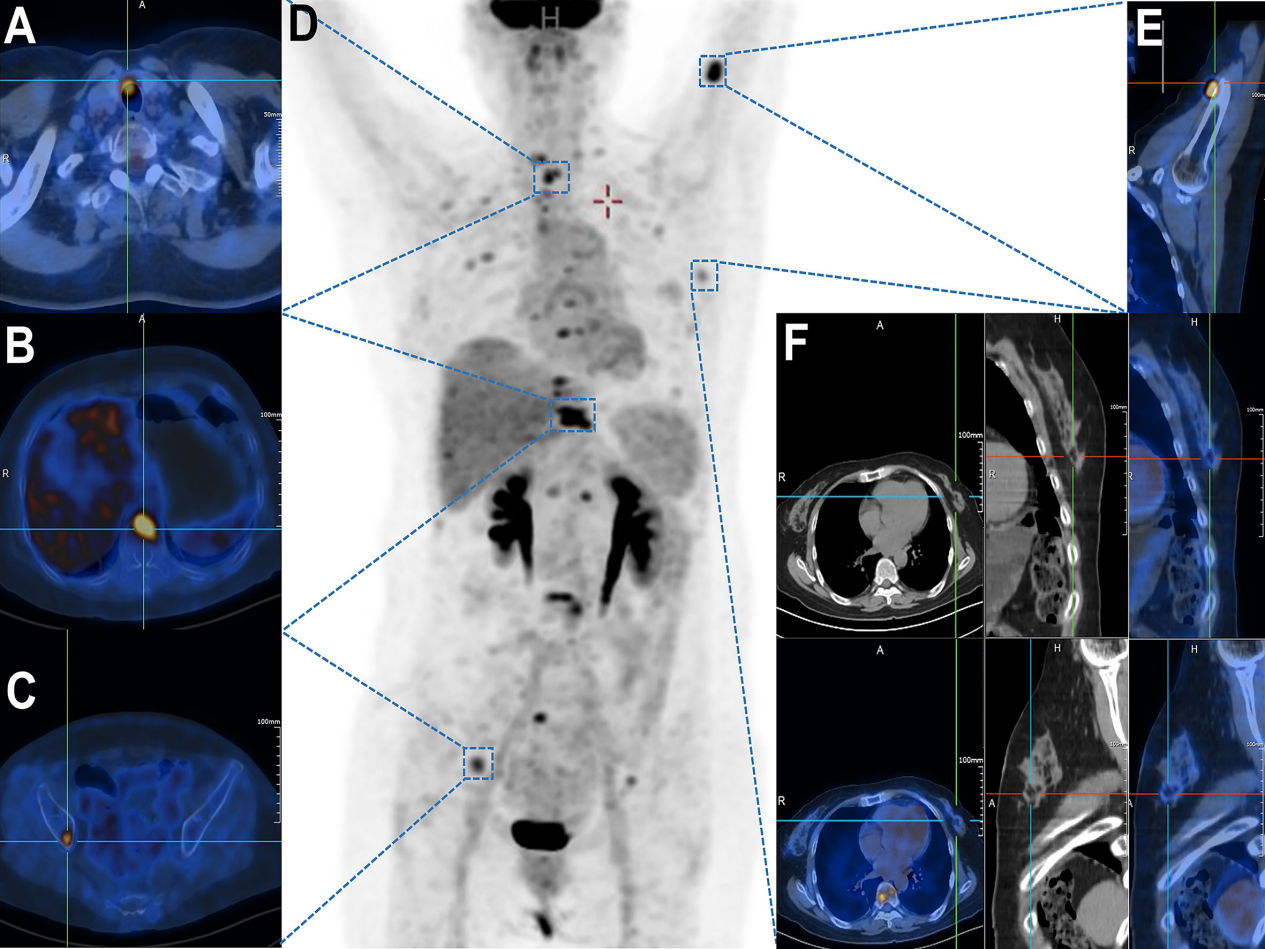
Whole-body ^18^F-FDG PET/CT demonstrating multifocal hypermetabolic lesions. (A) Thyroid isthmus lesion with focally increased FDG uptake (SUVmax = 9.41). (B) Vertebral metastasis at T10 level (SUVmax = 11.64). (C) Right iliac bone lesion showing elevated metabolic activity (SUVmax = [*value*]). (D) Maximum intensity projection (MIP) image revealing systemic dissemination of metabolically active metastases. (E) Left proximal humeral lesion with abnormal FDG avidity (SUVmax = [*value*]). (F) A suspicious mass in the left breast upper-outer quadrant demonstrated focal FDG avidity (SUVmax = 2.19) on axial, coronal, and sagittal fused PET/CT views.
